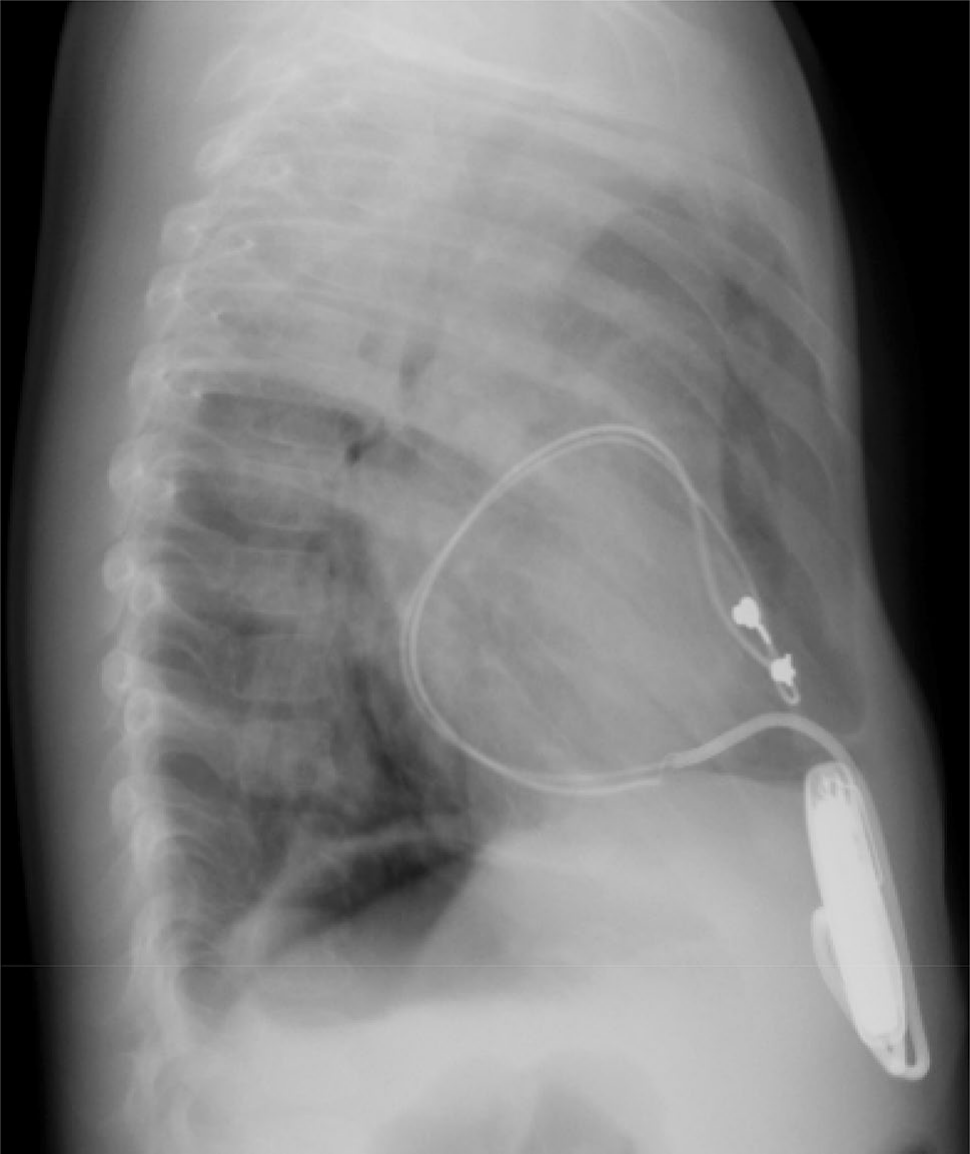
Lateral view of the X-ray which was taken at 11 months of age to diagnose pneumoniae in the other hospital
